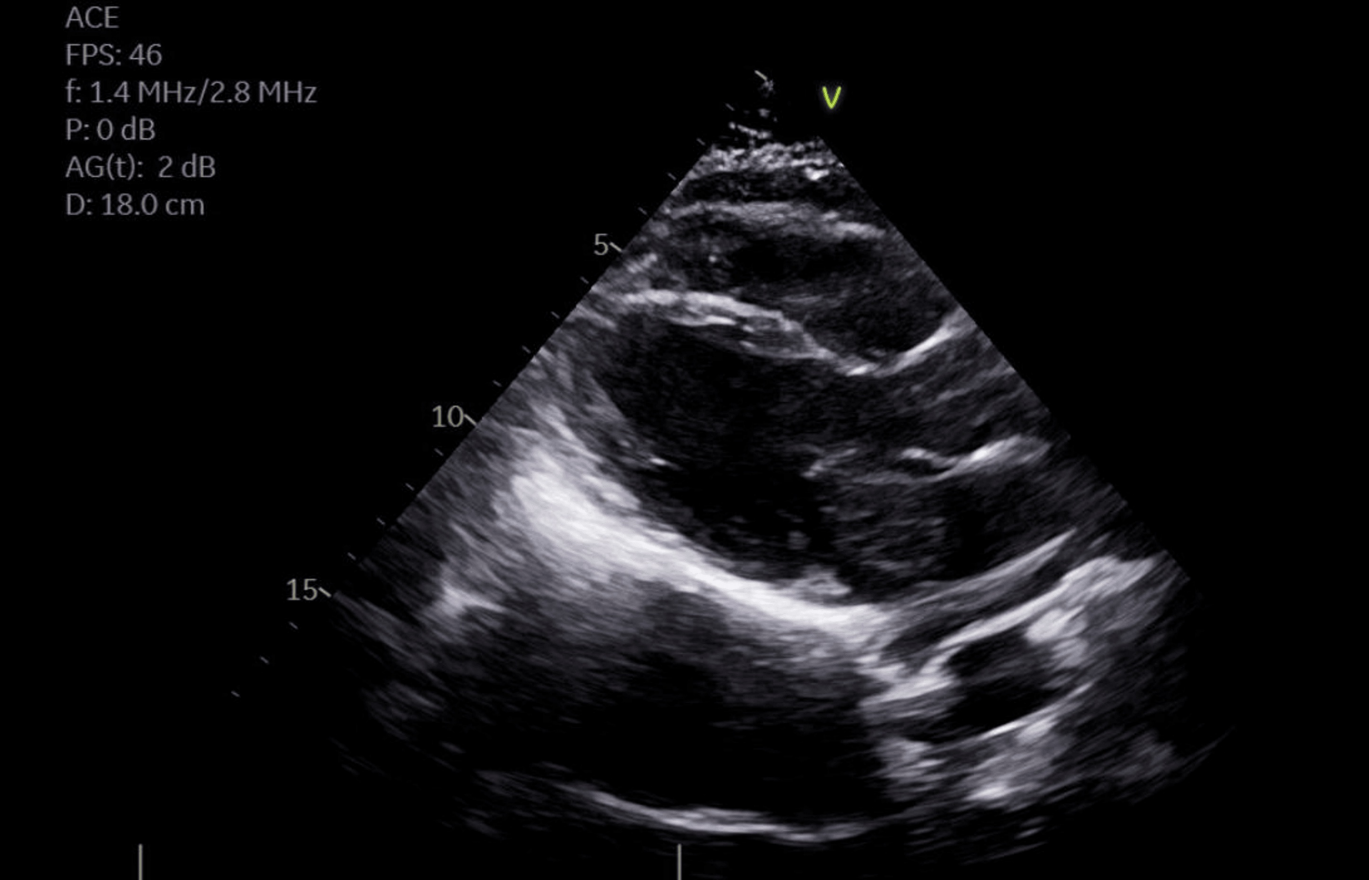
The transthoracic echocardiogram
